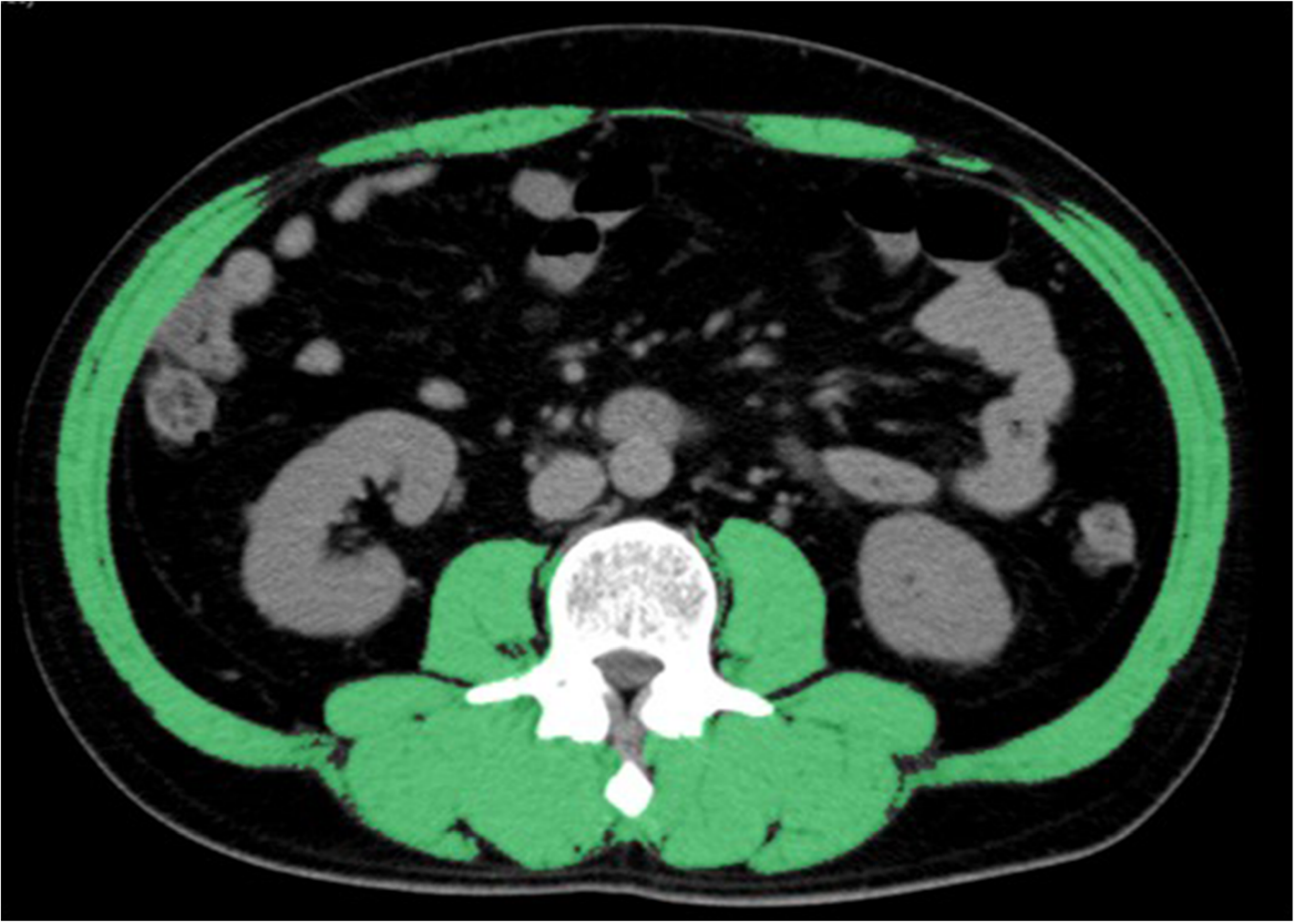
Measurement of SMM in RGC patients. Axial computed tomography slice of the third lumbar vertebra (L3). Green areas indicate skeletal muscle mass. Abbreviations: RGC, recurrent gastric cancer; SMM, skeletal muscle mass
